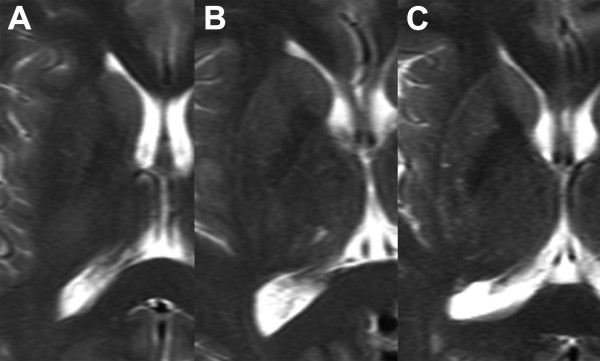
Axial T2-weighted images of normal subjects showing different degrees of conspicuity of HPR. HPR results classified as absent (A), vague (B), and present (C) are shown.
